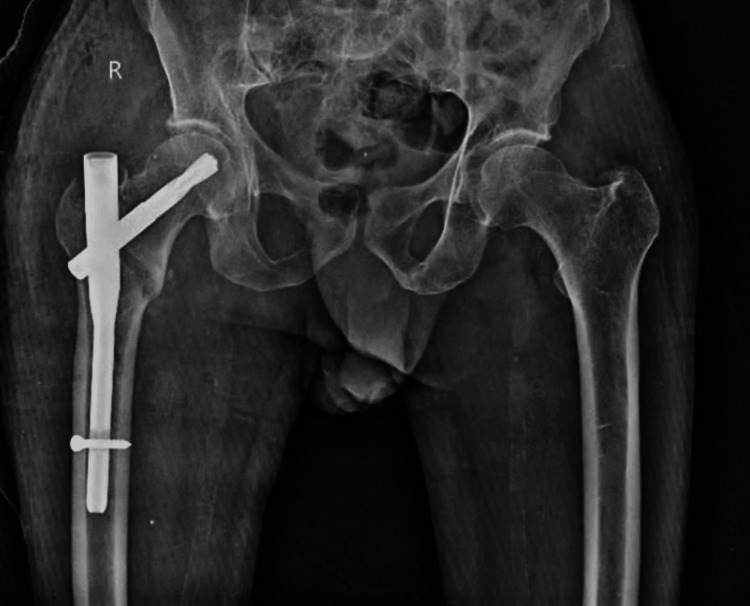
Post-operative x-ray of right femur.
